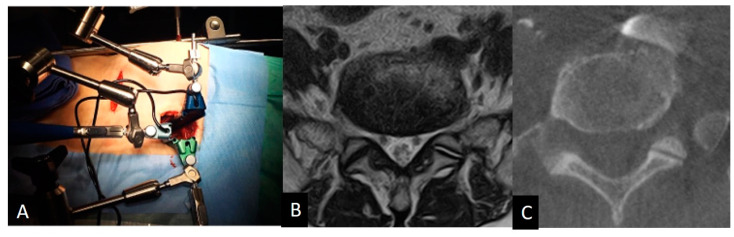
(A): OLIF51 surgery setup. A specially designed triple-arm retractor was used for safe retraction of vascular tissue, exposing the L5/S1 disc. (B) Preoperative MRI axial scan in the supine position at the L5 caudal endplate level. (C) Intraoperative enhanced CT scan in the right decubitus position at the L5 caudal endplate level.
